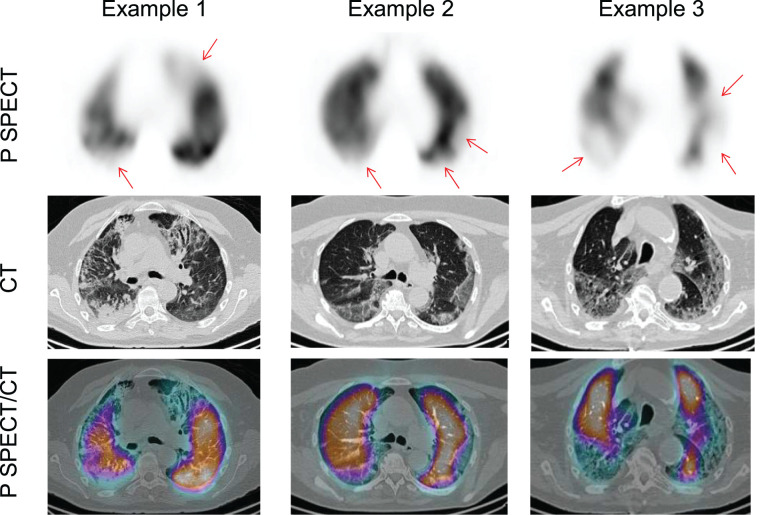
Examples of negative perfusion SPECT/CT results, with perfusion defects (arrows) matched with chest CT findings of COVID-19 disease. P = perfusion.
